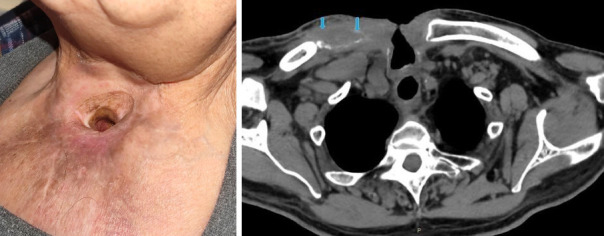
Postoperative at 6 months: clinical stability. Axial CT scan: fibrosis filling the clavicular resection space (blue arrows).
